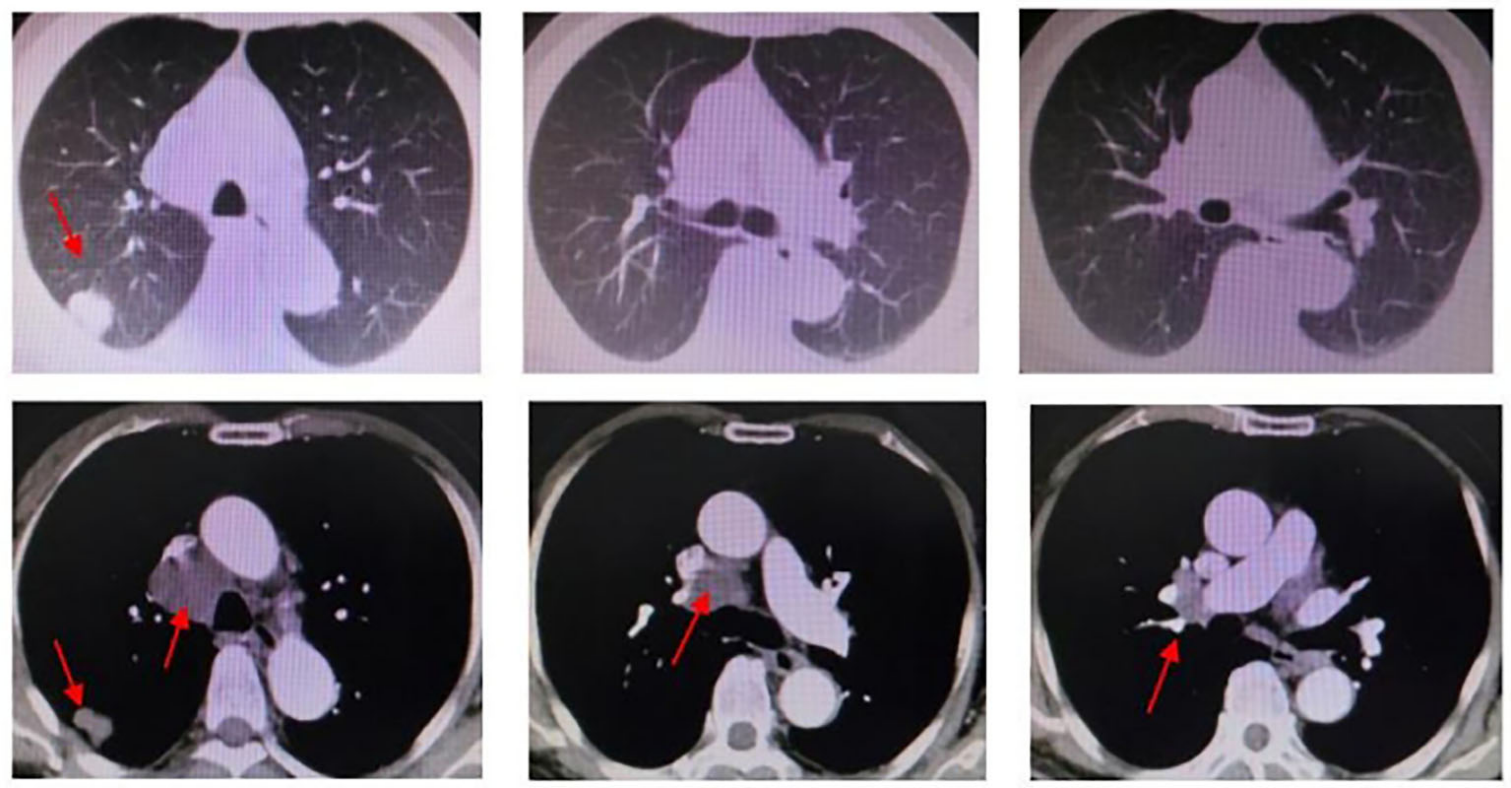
Chest CT (2022.06.27): right upper lobe and right hilar region masses, similar to the previous one. Mediastinal and right hilar lymph nodes metastasis; the larger one is approximately 3.9 * 4.1cm in diameter.
